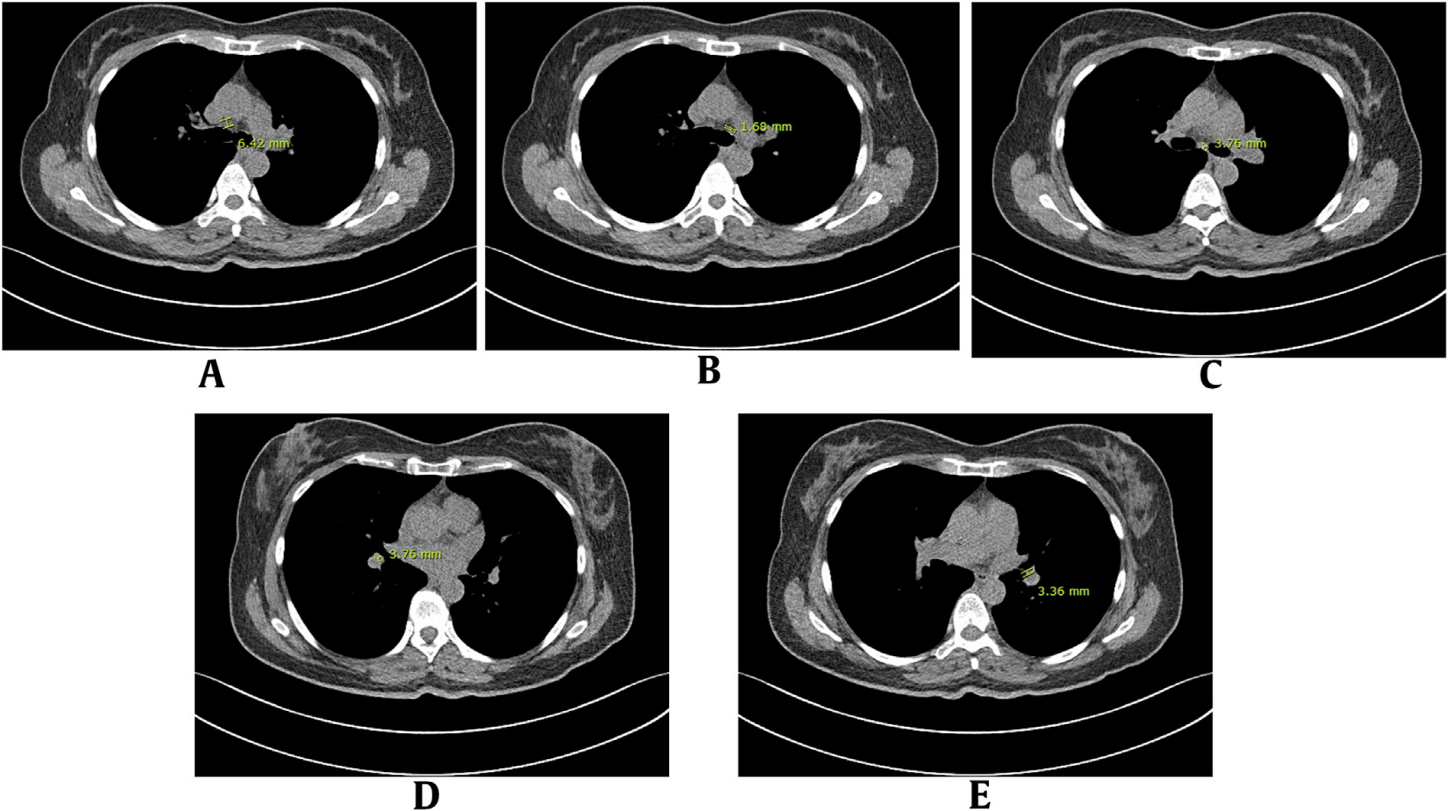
Methods of measuring short axis diameter of lymph nodes on CT image. (A) The right lower paratracheal or right hilar, (B) left lower paratracheal or left hilar, (C) subcarinal, (D) right inferior, and (E) left inferior interlobar lymph nodes were measured at the largest, most distinct location with minimal motion artifacts. CT, computed tomography.
